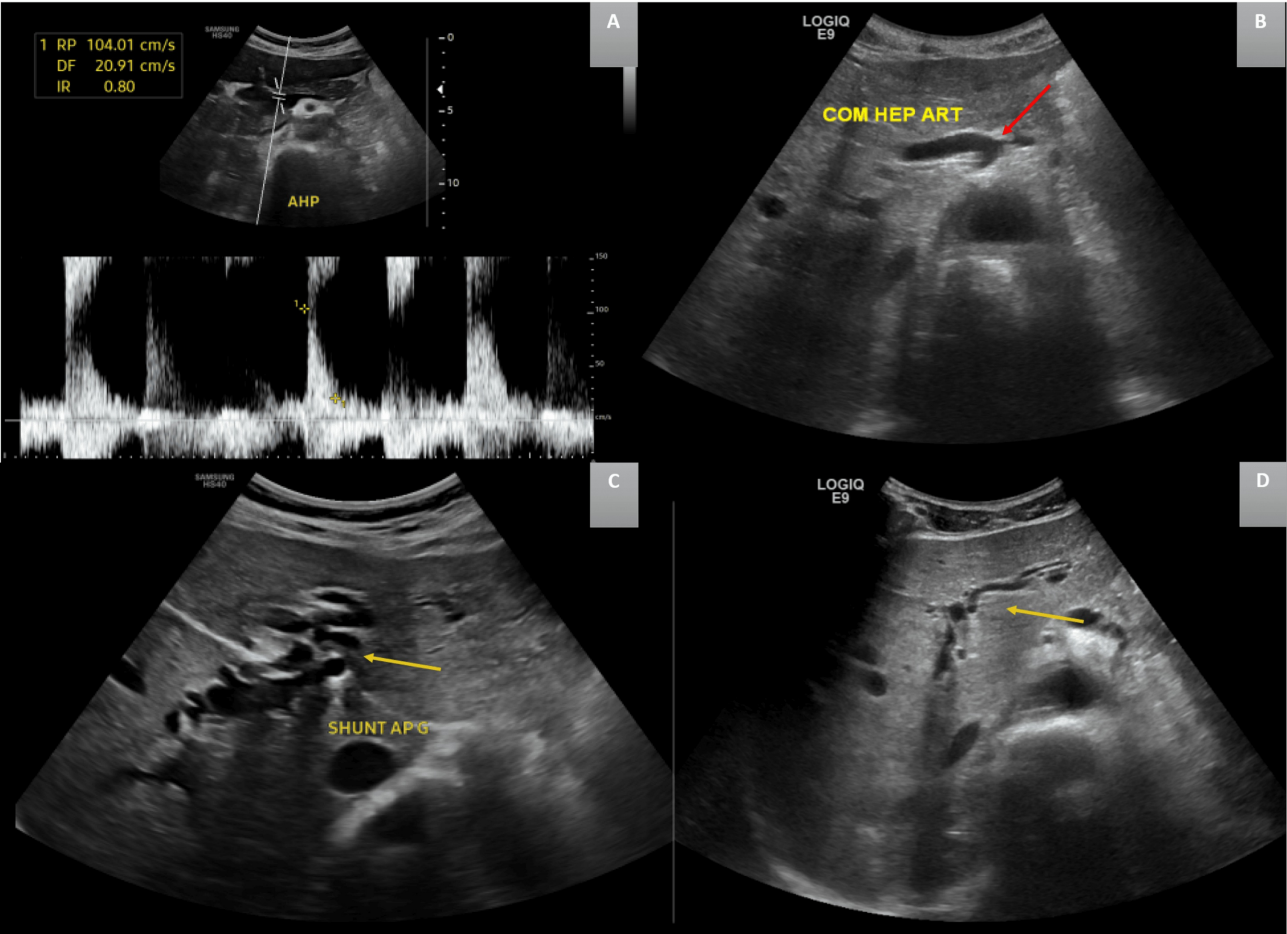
Ultrasound images (A) Color Doppler revealing high velocity (104 cm/second) and a high resistance index in the hepatic artery measuring 0.8; (B) Dilation of the hepatic artery (red arrow); (C, D) Tortuous intrahepatic tubular structures and arterio-portal shunts (yellow arrows)
